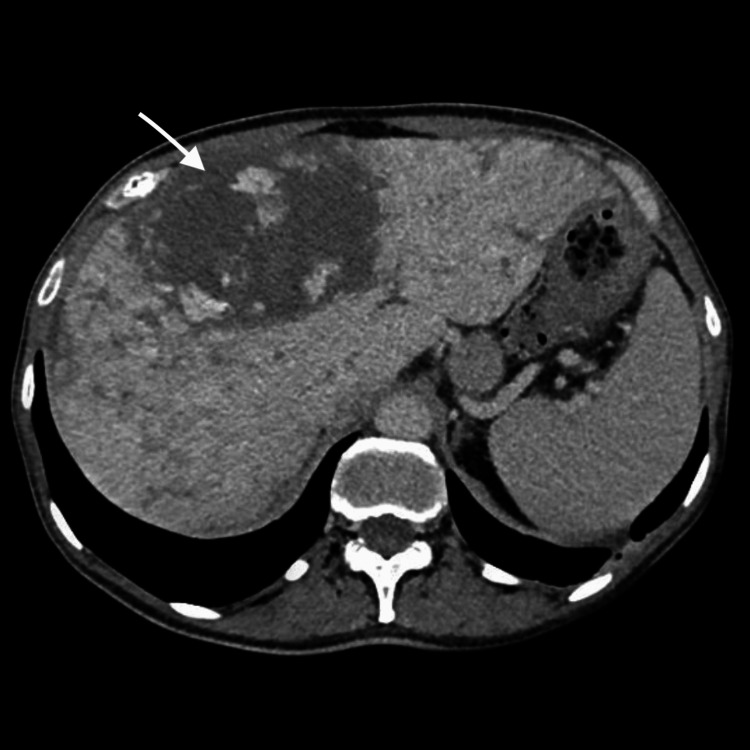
Axial contrast-enhanced CT scan of the abdomen in the portal venous phase (Hospital day 45). Moderate hepatomegaly with lobulated contours and diffusely heterogenous structure with a diffuse mottled appearance continues to be seen. Multiple nodular foci of various sizes can be seen, corresponding to previously known hemangiomas, the largest measuring approximately 86 x 75 mm in segment IV.
